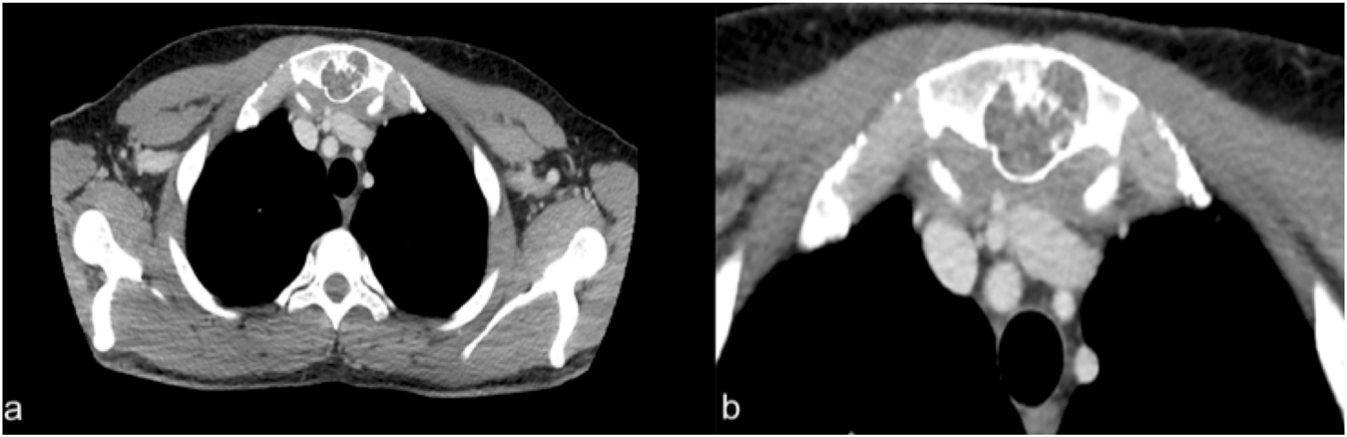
Sternal enchondroma in a 66-year-old male patient. a Axial CT image in mediastinal window shows a lytic lesion with defined margins involving the manubrium sterni. b More cranial axial CT slice confirms the cartilaginous nature of the lesion, with absence of aggressive features such as soft tissue extension or cortical disruption
